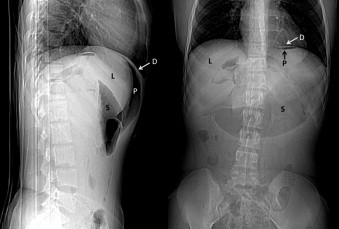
CT scout films of the abdomen demonstrating a pneumopertioneum (P) that is contained by the diaphregram (D) and the liver (L). A dilated stomach (S) is also seen.
